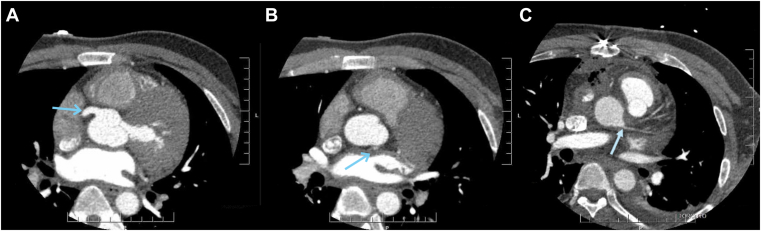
Coronary CT showing (A) ostium of a dominant RCA (arrow) emerging from the right coronary cusp. (B) Left coronary cusp with no distinct lumen connected to the left main (arrow). The left main coronary artery is small in caliber. (C) CT coronary performed after left main ostioplasty demonstrating connection of the left main coronary artery to the aorta (arrow). CT, computed tomography; RCA, right coronary artery.
